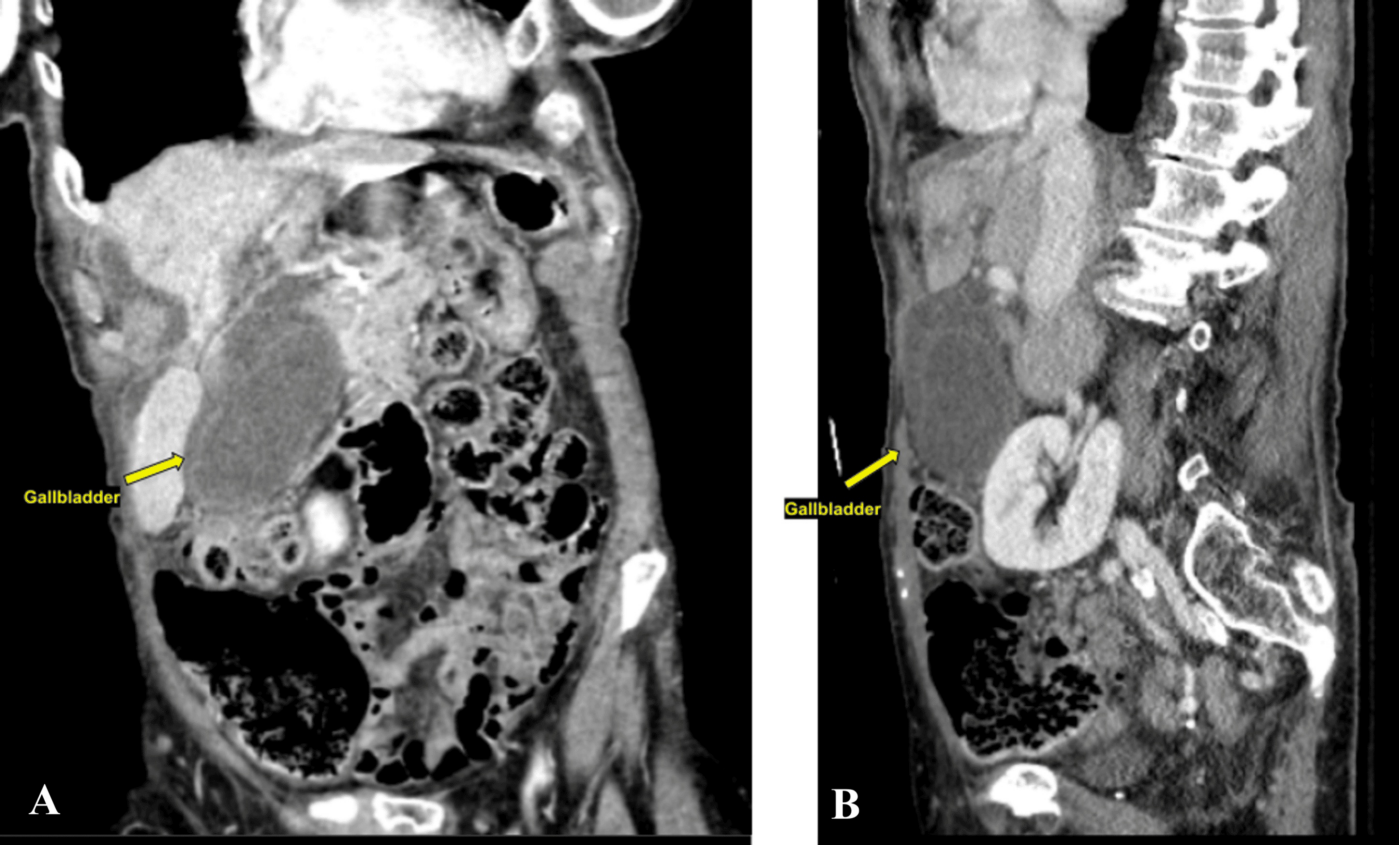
CT scan with IV contrast demonstrating a markedly distended gallbladder with wall thickening, pericholecystic fluid and fat stranding (left: coronal view, right: sagittal view). (A) axial view of computed tomography, arrow indicates the distended gallbladder. (B) sagittal view of computed tomography, arrow indicates the distended gallbladder. The classic findings (“whirl sign,” “floating gallbladder,” etc.) were not seen.
